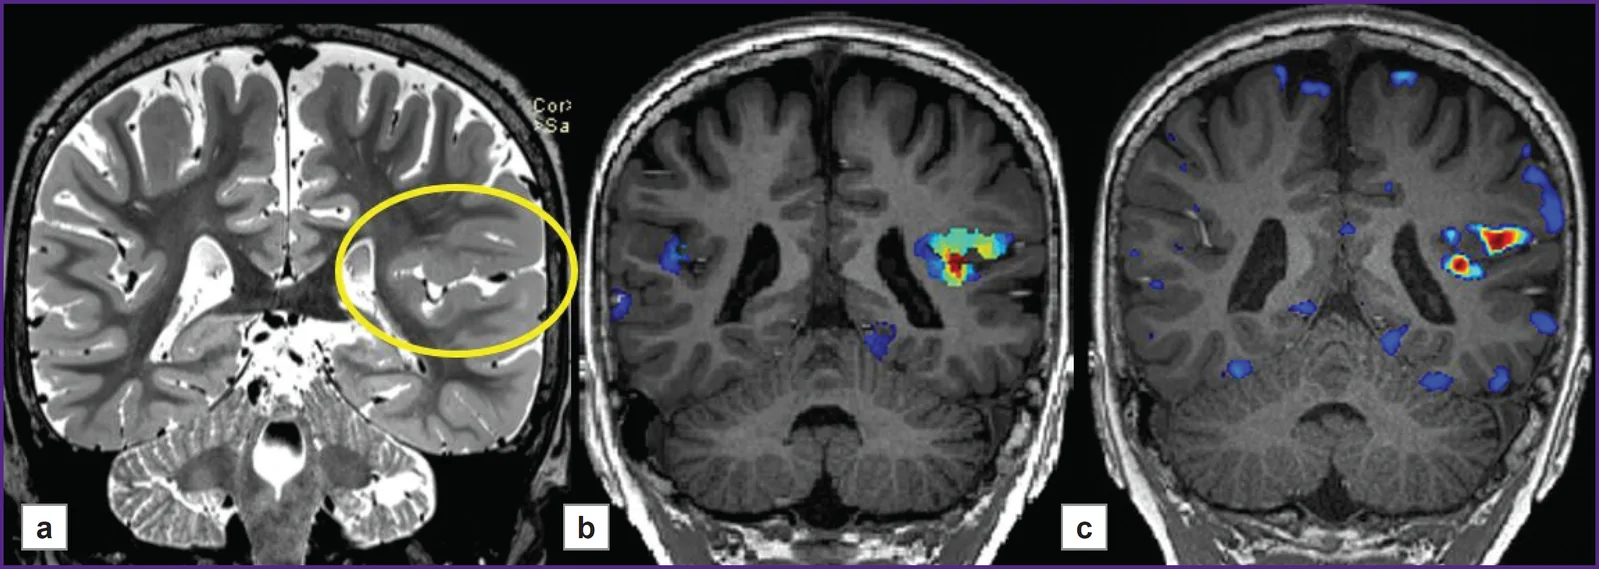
MRI of a 10-year-old boy with focal cortical dysplasia in the left temporo-insular region: (a) standard MRI: no visible abnormalities (highlighted in an oval); (b) classical morphometry: a zone of disrupted demarcation; (c) multimodal morphometry: confirmation of the affected zone
